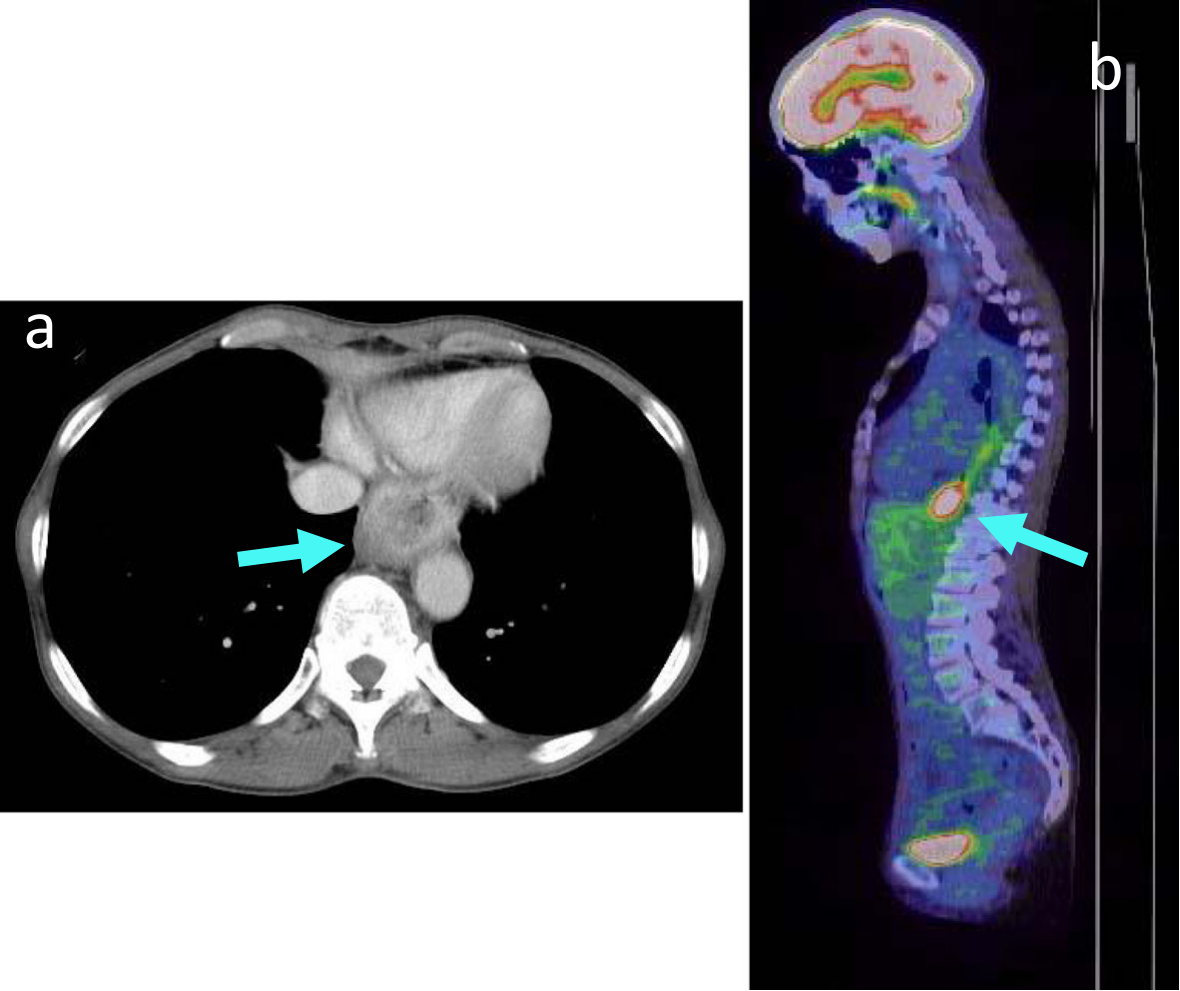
CT and PET/CT 1 year and 6 months later. a CT scan found the obvious wall thickening at the lower esophagus (arrows). b PET/CT showed increased FDG uptake in the lower esophagus (SUV max, 9.5) (arrows)
